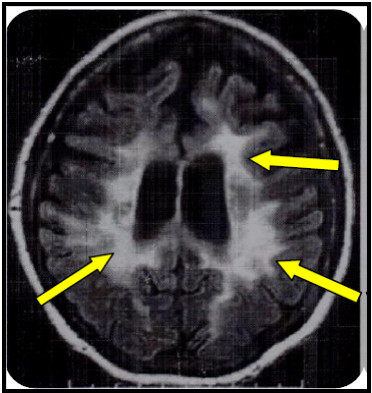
Brain MRI of the patient with adult form of MLD 'arrows' indicate the lesions of the white matter.
